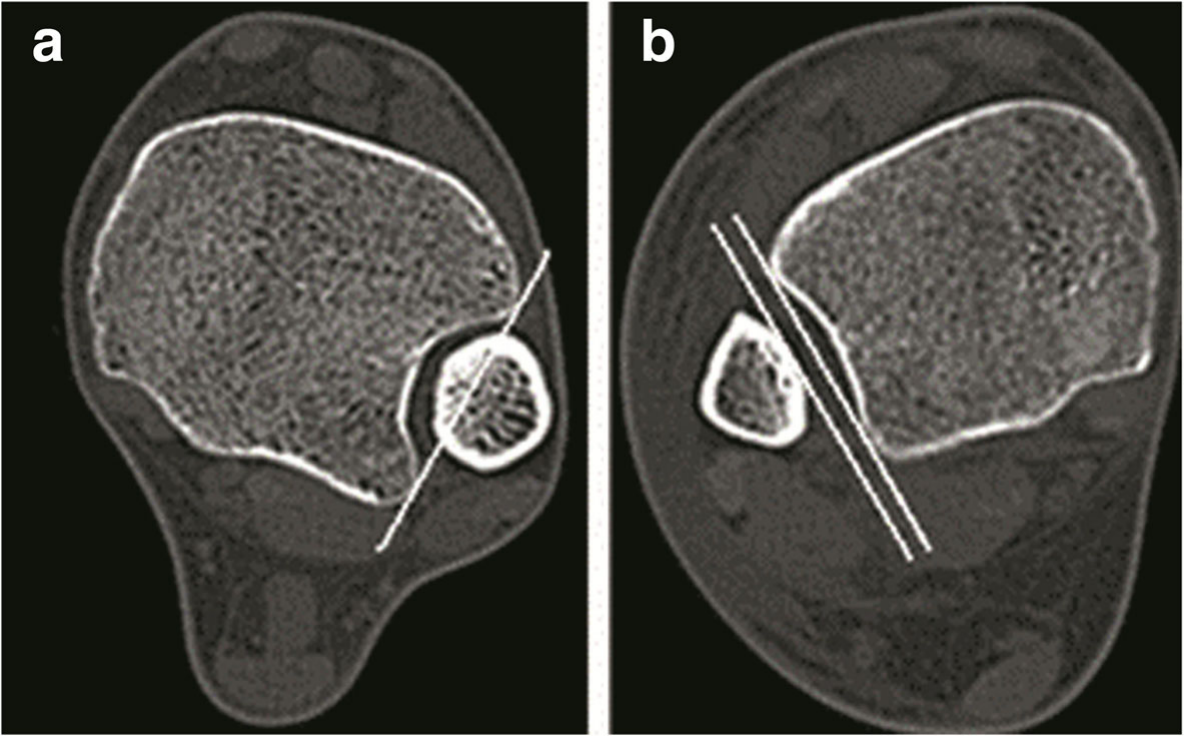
CT scans of representative patients with a contained-type and b separated-type DTS. a The fibula was contained in the fibular incisure. b The X-ray can penetrate through the interspace between the fibula and the fibular incisure
